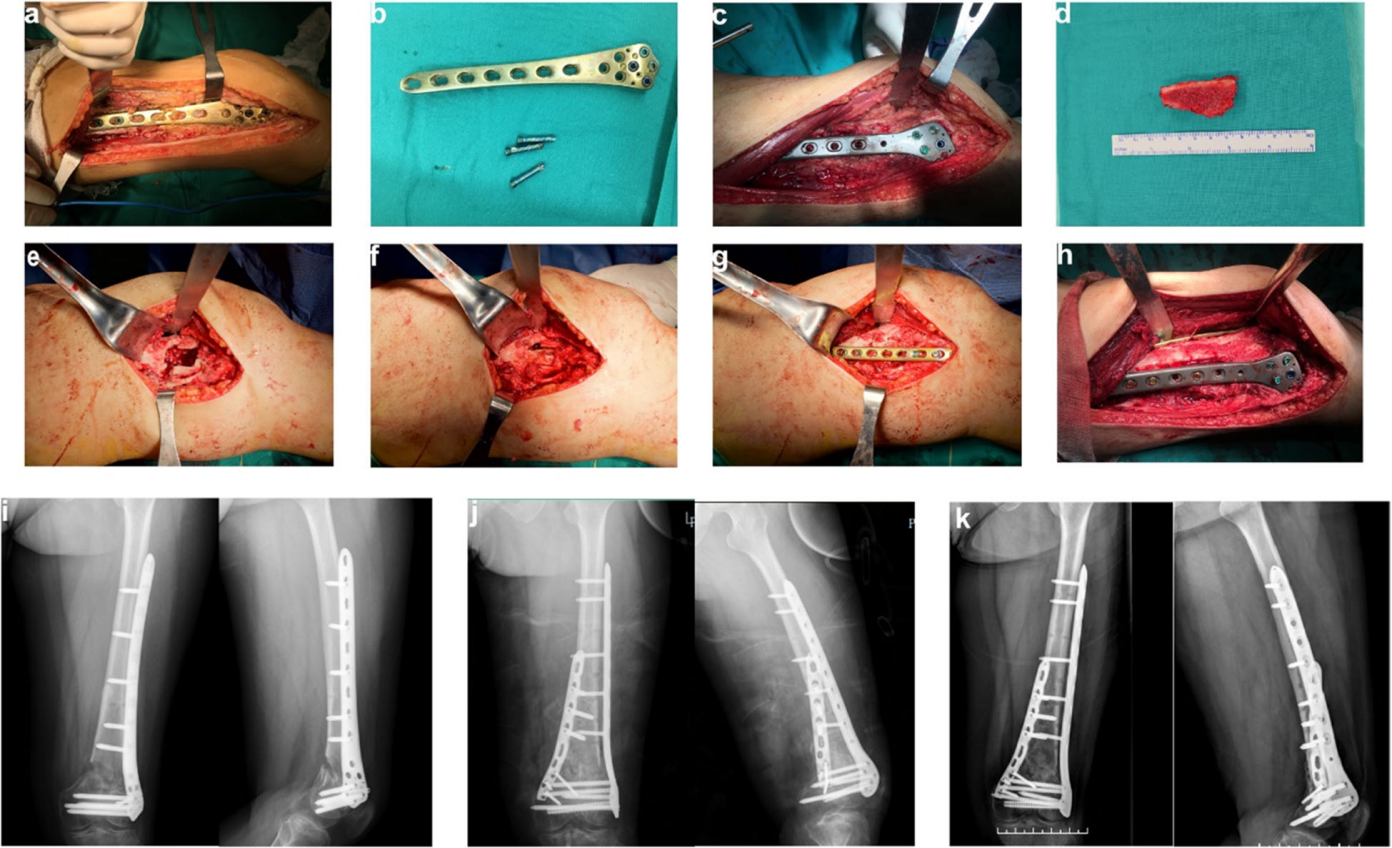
Surgical procedure and radiographic findings of a 39-year-old female patient. a The original internal fixation device is exposed. b The original internal fixation device is removed. c The lateral plate is fixed on the outside of the femur. d Image shows the J-bone graft before press-fit insertion. e Image shows the fenestration in the non-union of medial distal femur. f Image shows the tap J-bone graft on the medial side of the distal femur. g Image shows the medial plate protection J-bone. h The DP is grafted with J-bone. i Non-union is seen 10 months following surgery. j The plate is exchanged and grafted with a J-bone. k Three months following grafting, union is achieved. *DP* double-plate
